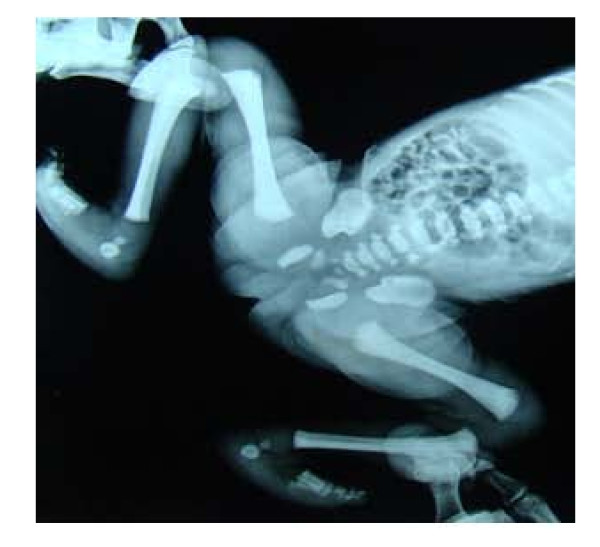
Rocker bottom feet visible at birth -visualized in the X-ray, during the retrospective study.
